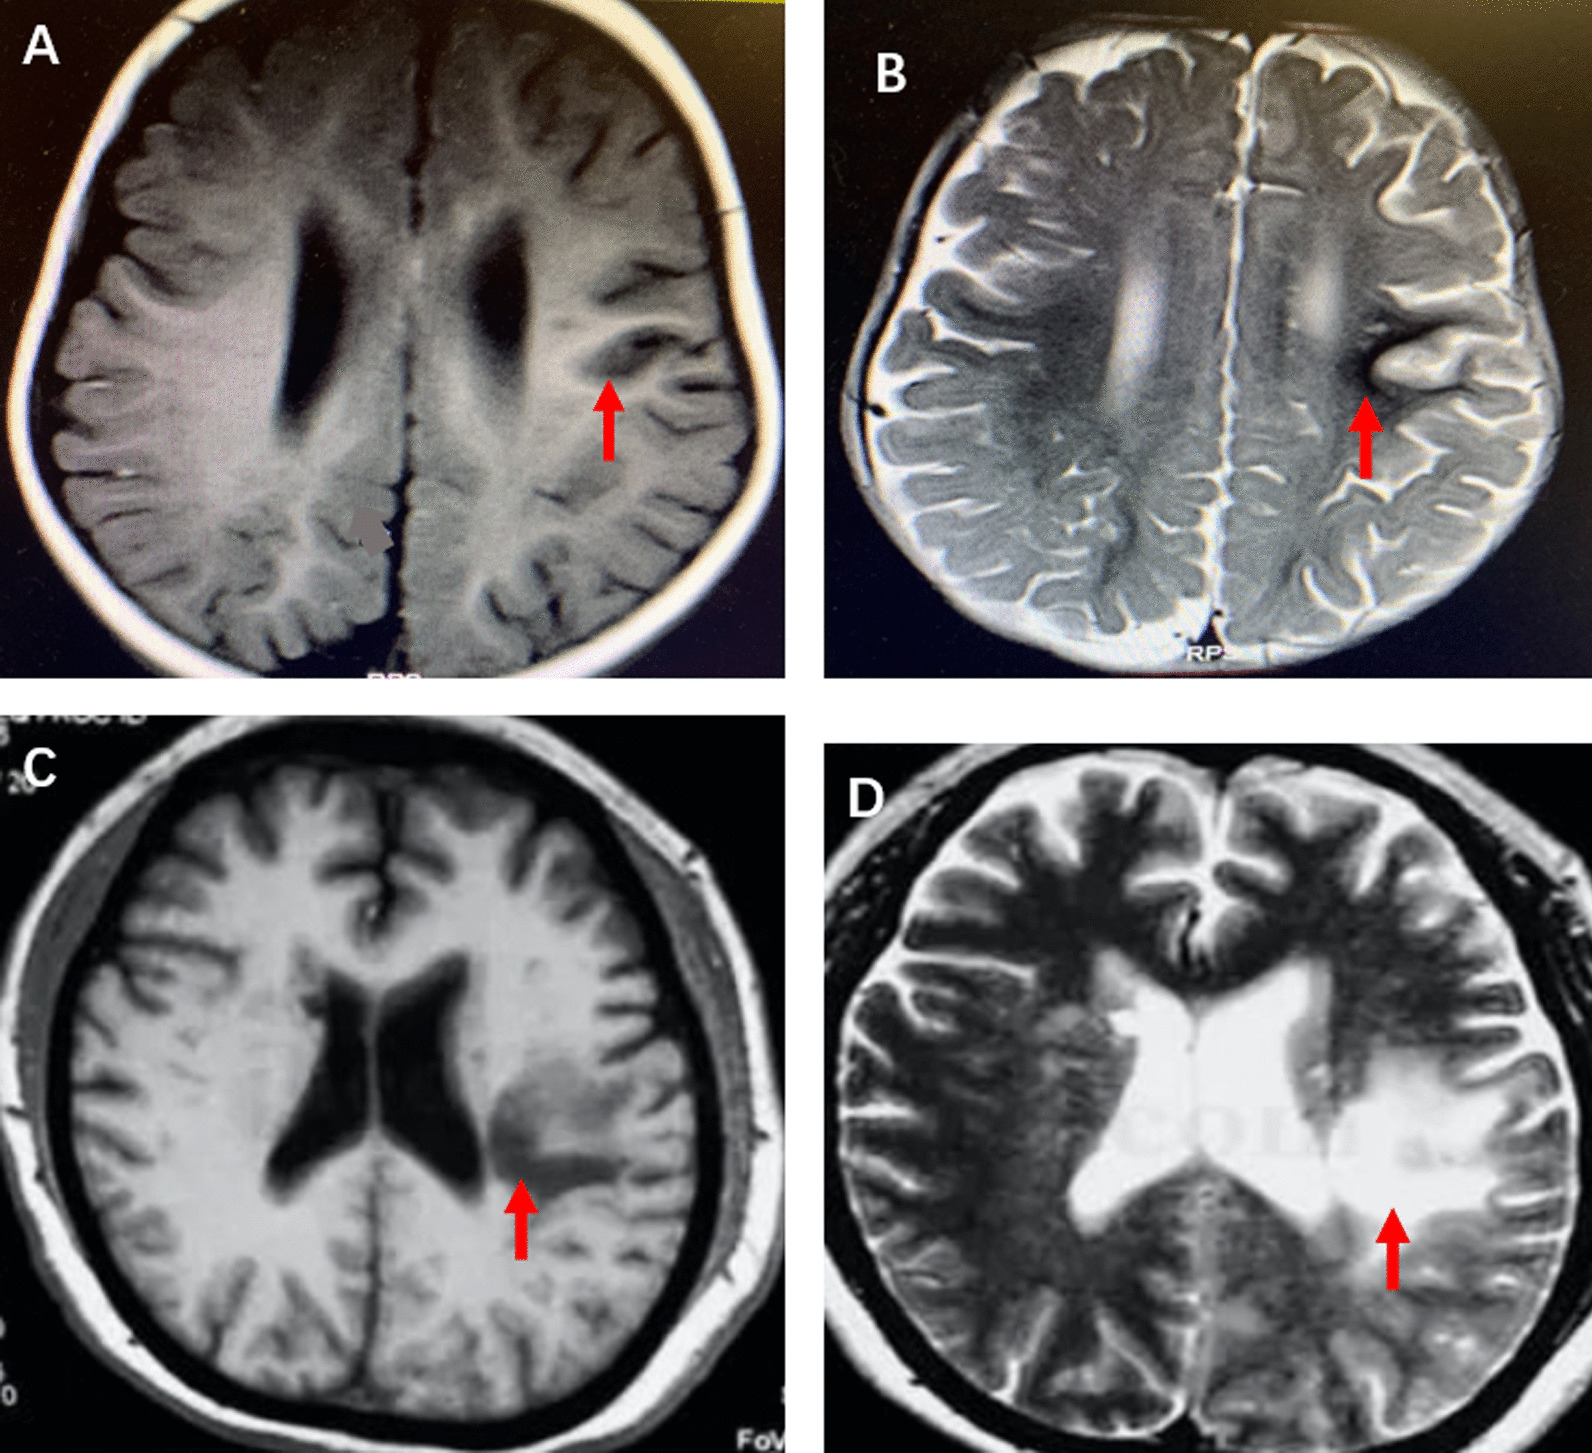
Cranial MRI showing cerebral infarction. The areas of restricted diffusion compatible with hypointense infarction in the left temporal area (A) and hyperintense infarction on apparent diffusion coefficient mapping (B). New large cerebral infarction in the left hemisphere [T1-WI (C) and T2-WI (D)]. Arrows show the areas of cerebral infarction
